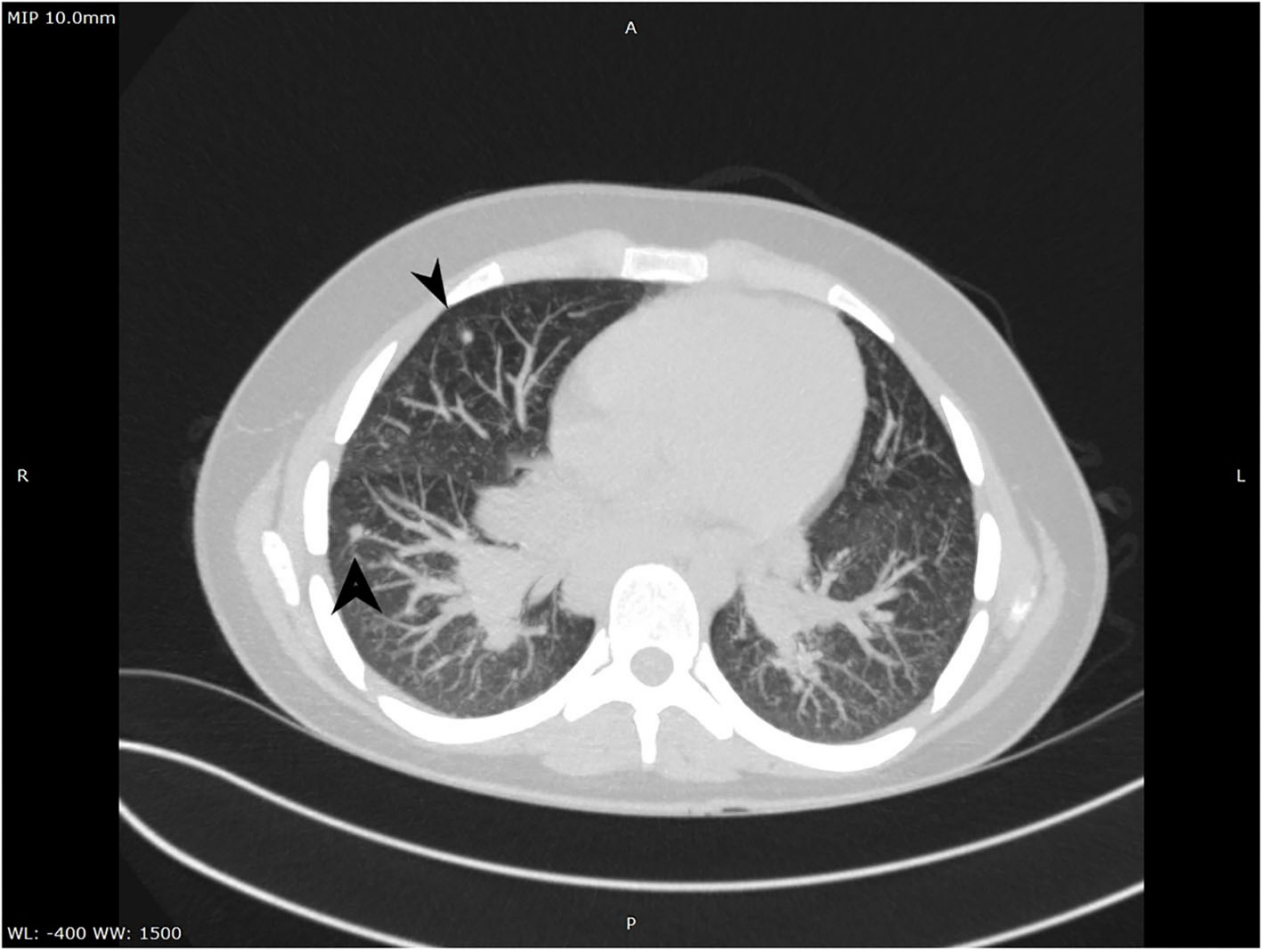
CT aspect of lung micronodules.
